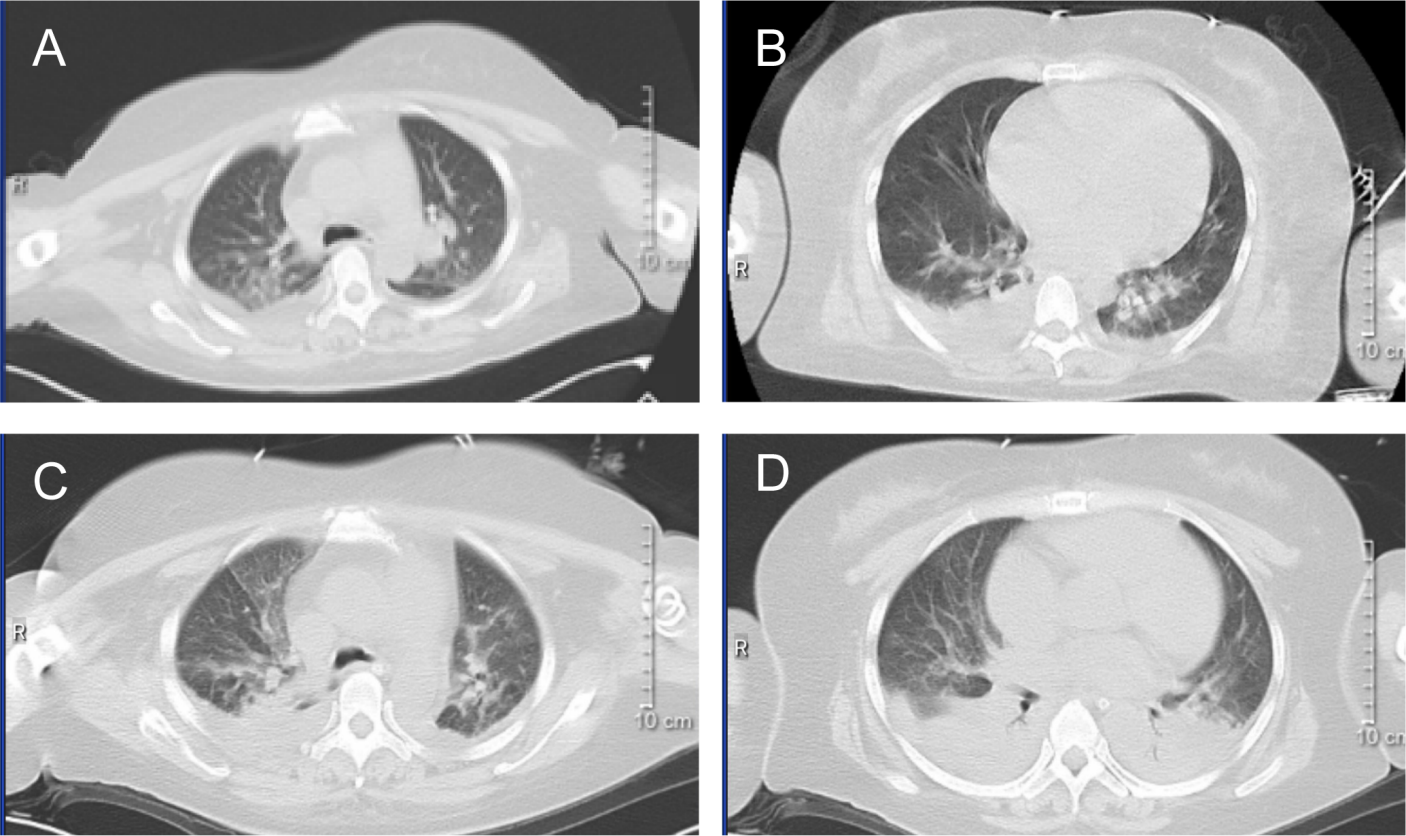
CT image. (A, B) the chest CT image of February 28, 2024. (C, D) the chest CT image of March 3, 2024.
